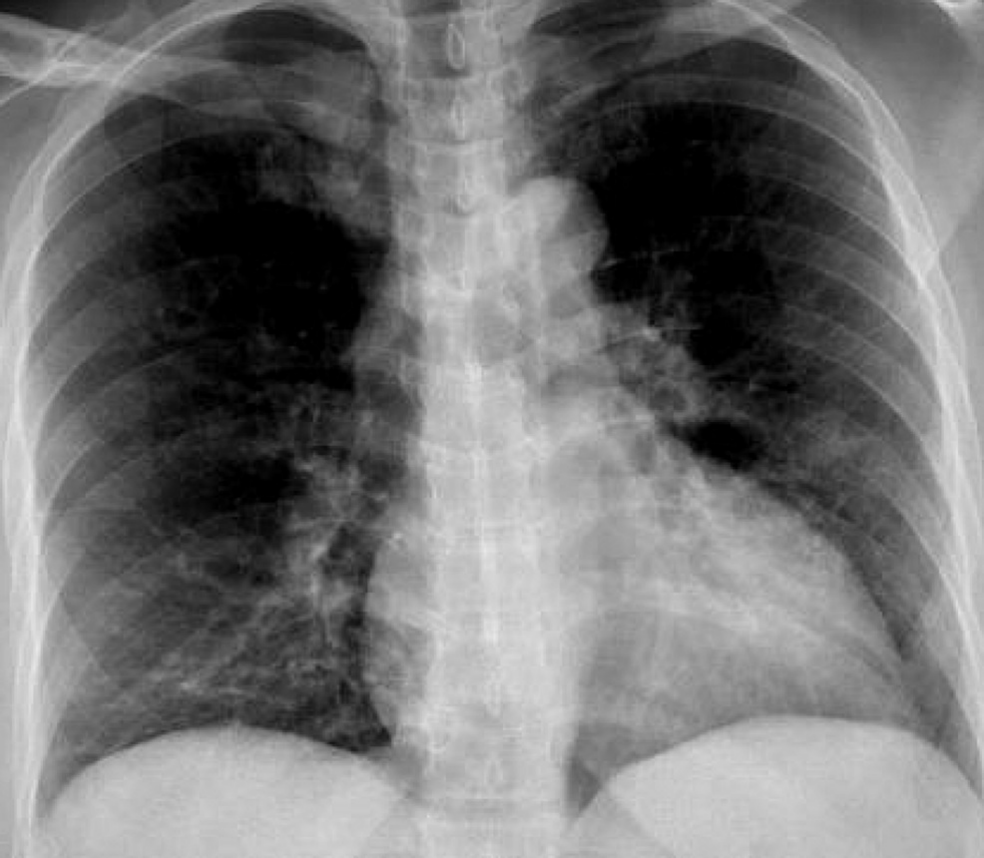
Chest X-ray showing subtle alveolar opacities in left midlung, linear opacities at the bases.
